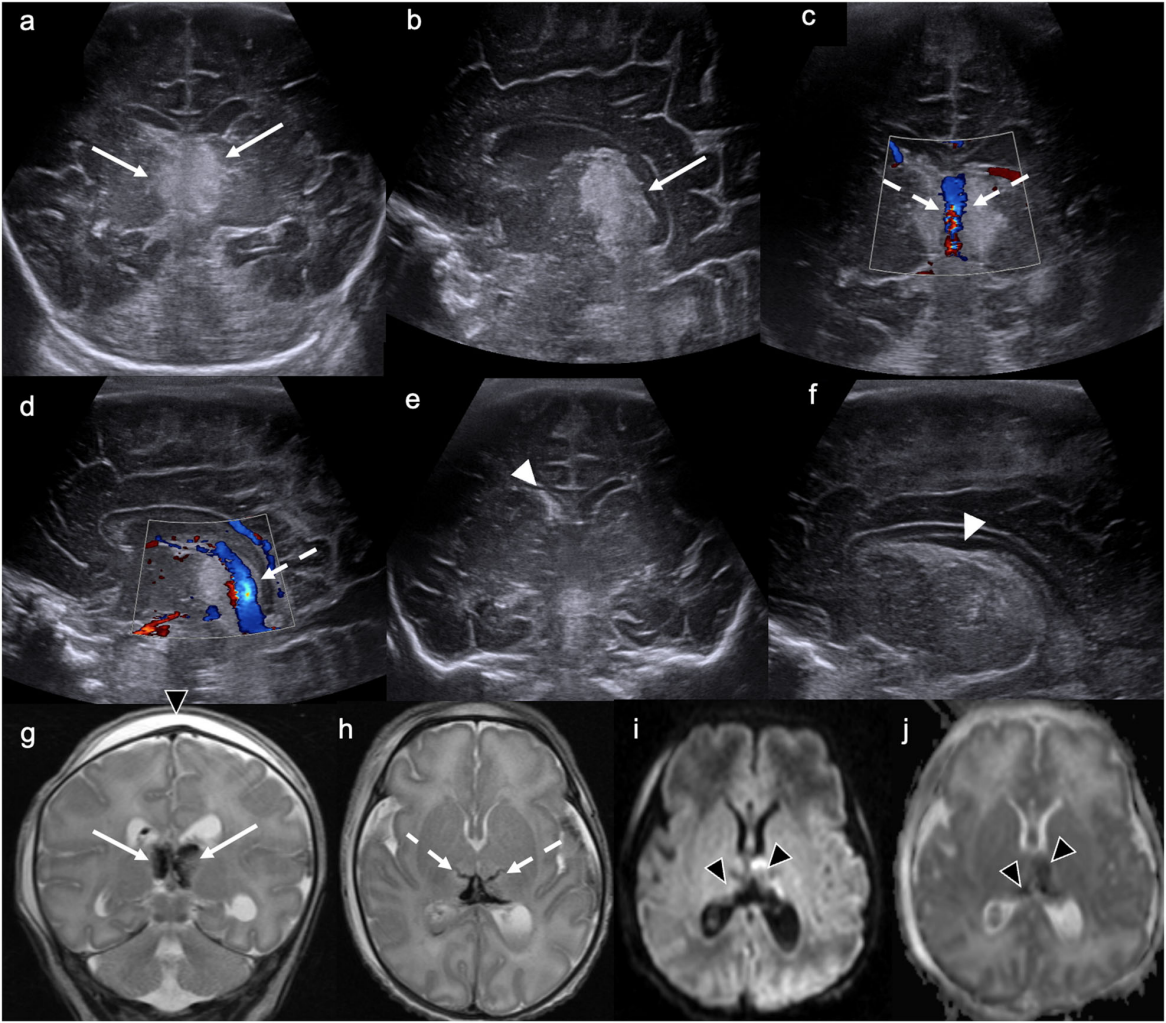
Cerebral sinovenous thrombosis. Day 2 ultrasound in a 36-week gestation neonate with difficulty feeding after a complicated (failed forceps) delivery. a Coronal and (b) left parasagittal images demonstrate bilateral, slightly asymmetrical (left > right) increased echogenicity of the medial thalami (solid arrows). c Coronal and (d) left parasagittal colour Doppler images demonstrate flow in the internal cerebral veins (dashed arrows), suggesting patency. e Coronal image demonstrates echogenic material in the right frontal horn, partially effacing it (white arrowhead). f Right parasagittal image confirms echogenic material in the caudothalamic groove (white arrowhead), consistent with germinal matrix haemorrhage. This constellation of findings—bilateral thalamic increased echogenicity, presumed haemorrhagic infarction, and right grade 1 intraventricular haemorrhage—is suggestive of cerebral sinovenous thrombosis. g Coronal T2WI demonstrates corresponding bilateral hypointensity in the medial thalami, confirming haemorrhage (solid arrows). Note the subgaleal collection secondary to traumatic delivery (black arrowhead). h Axial T2WI demonstrates linear low signal in bilateral perforator veins of the thalamus, consistent with thrombosis (dashed arrows), which was not evident on CUS. i Axial trace DWI and (j) ADC map demonstrate restricted diffusion in bilateral thalami (left > right) (black arrowheads), consistent with acute thalamic haemorrhagic venous infarction
